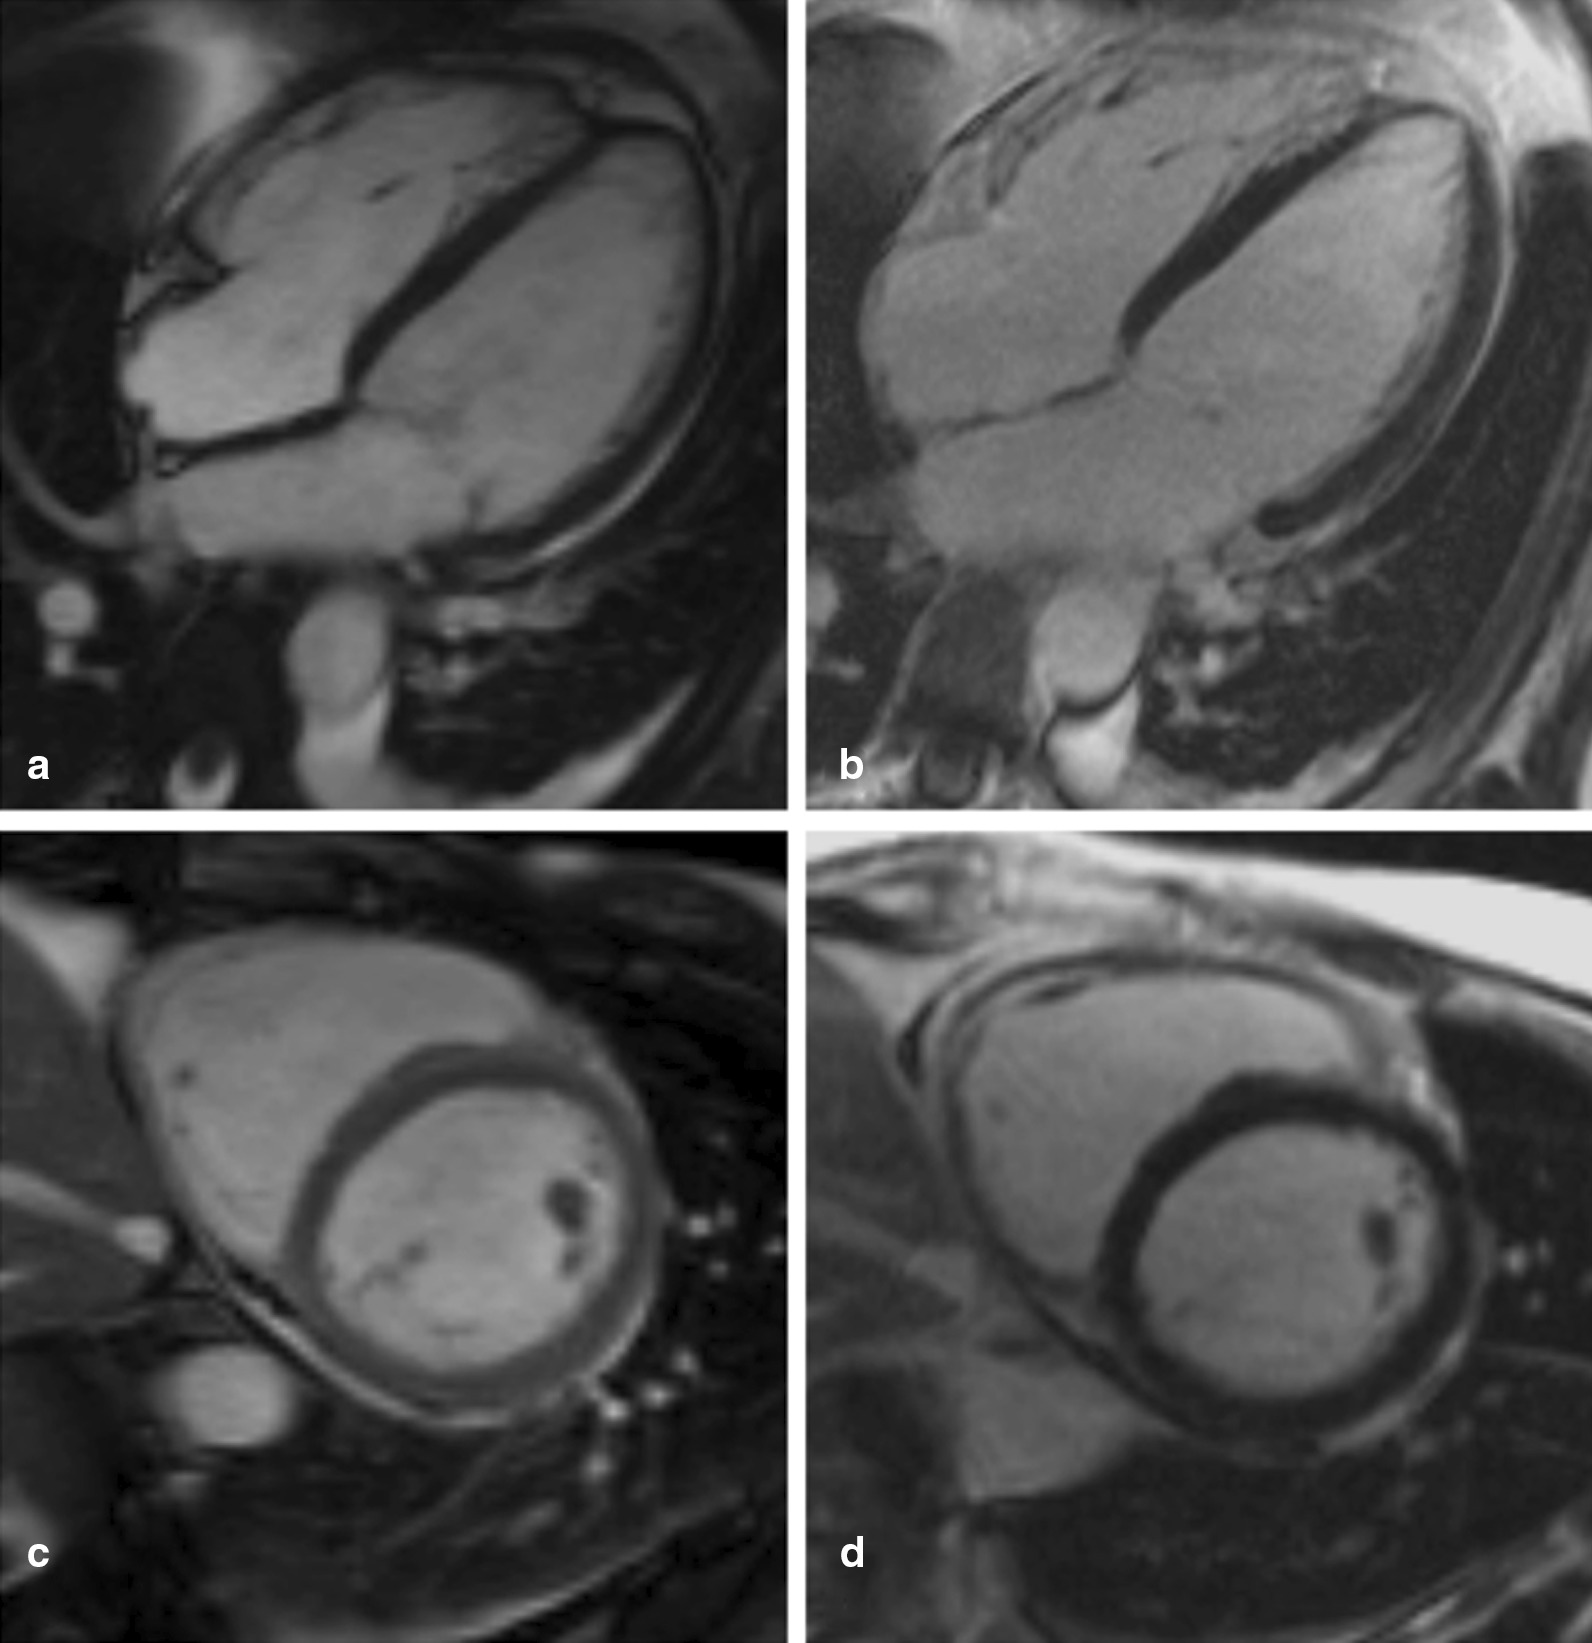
Cardiac MRI 3 weeks after admission shows 4 chamber views (a, b) and short axis views (c, d) in a functional test (cine MRI, a, c) and late enhancement (b, d), respectively, mildly enlarged systolic and diastolic volumes, a borderline reduced systolic LV function without myocardial late gadolinium enhancement (LV ejection fraction 56%, end-diastolic volume 87 ml/m^2^, end-systolic volume 38 ml/m^2^, stroke volume 48 ml/m^2^). Cardiac MRI images showed a minimal percicardial effusion and no evidence of myocardial oedema
